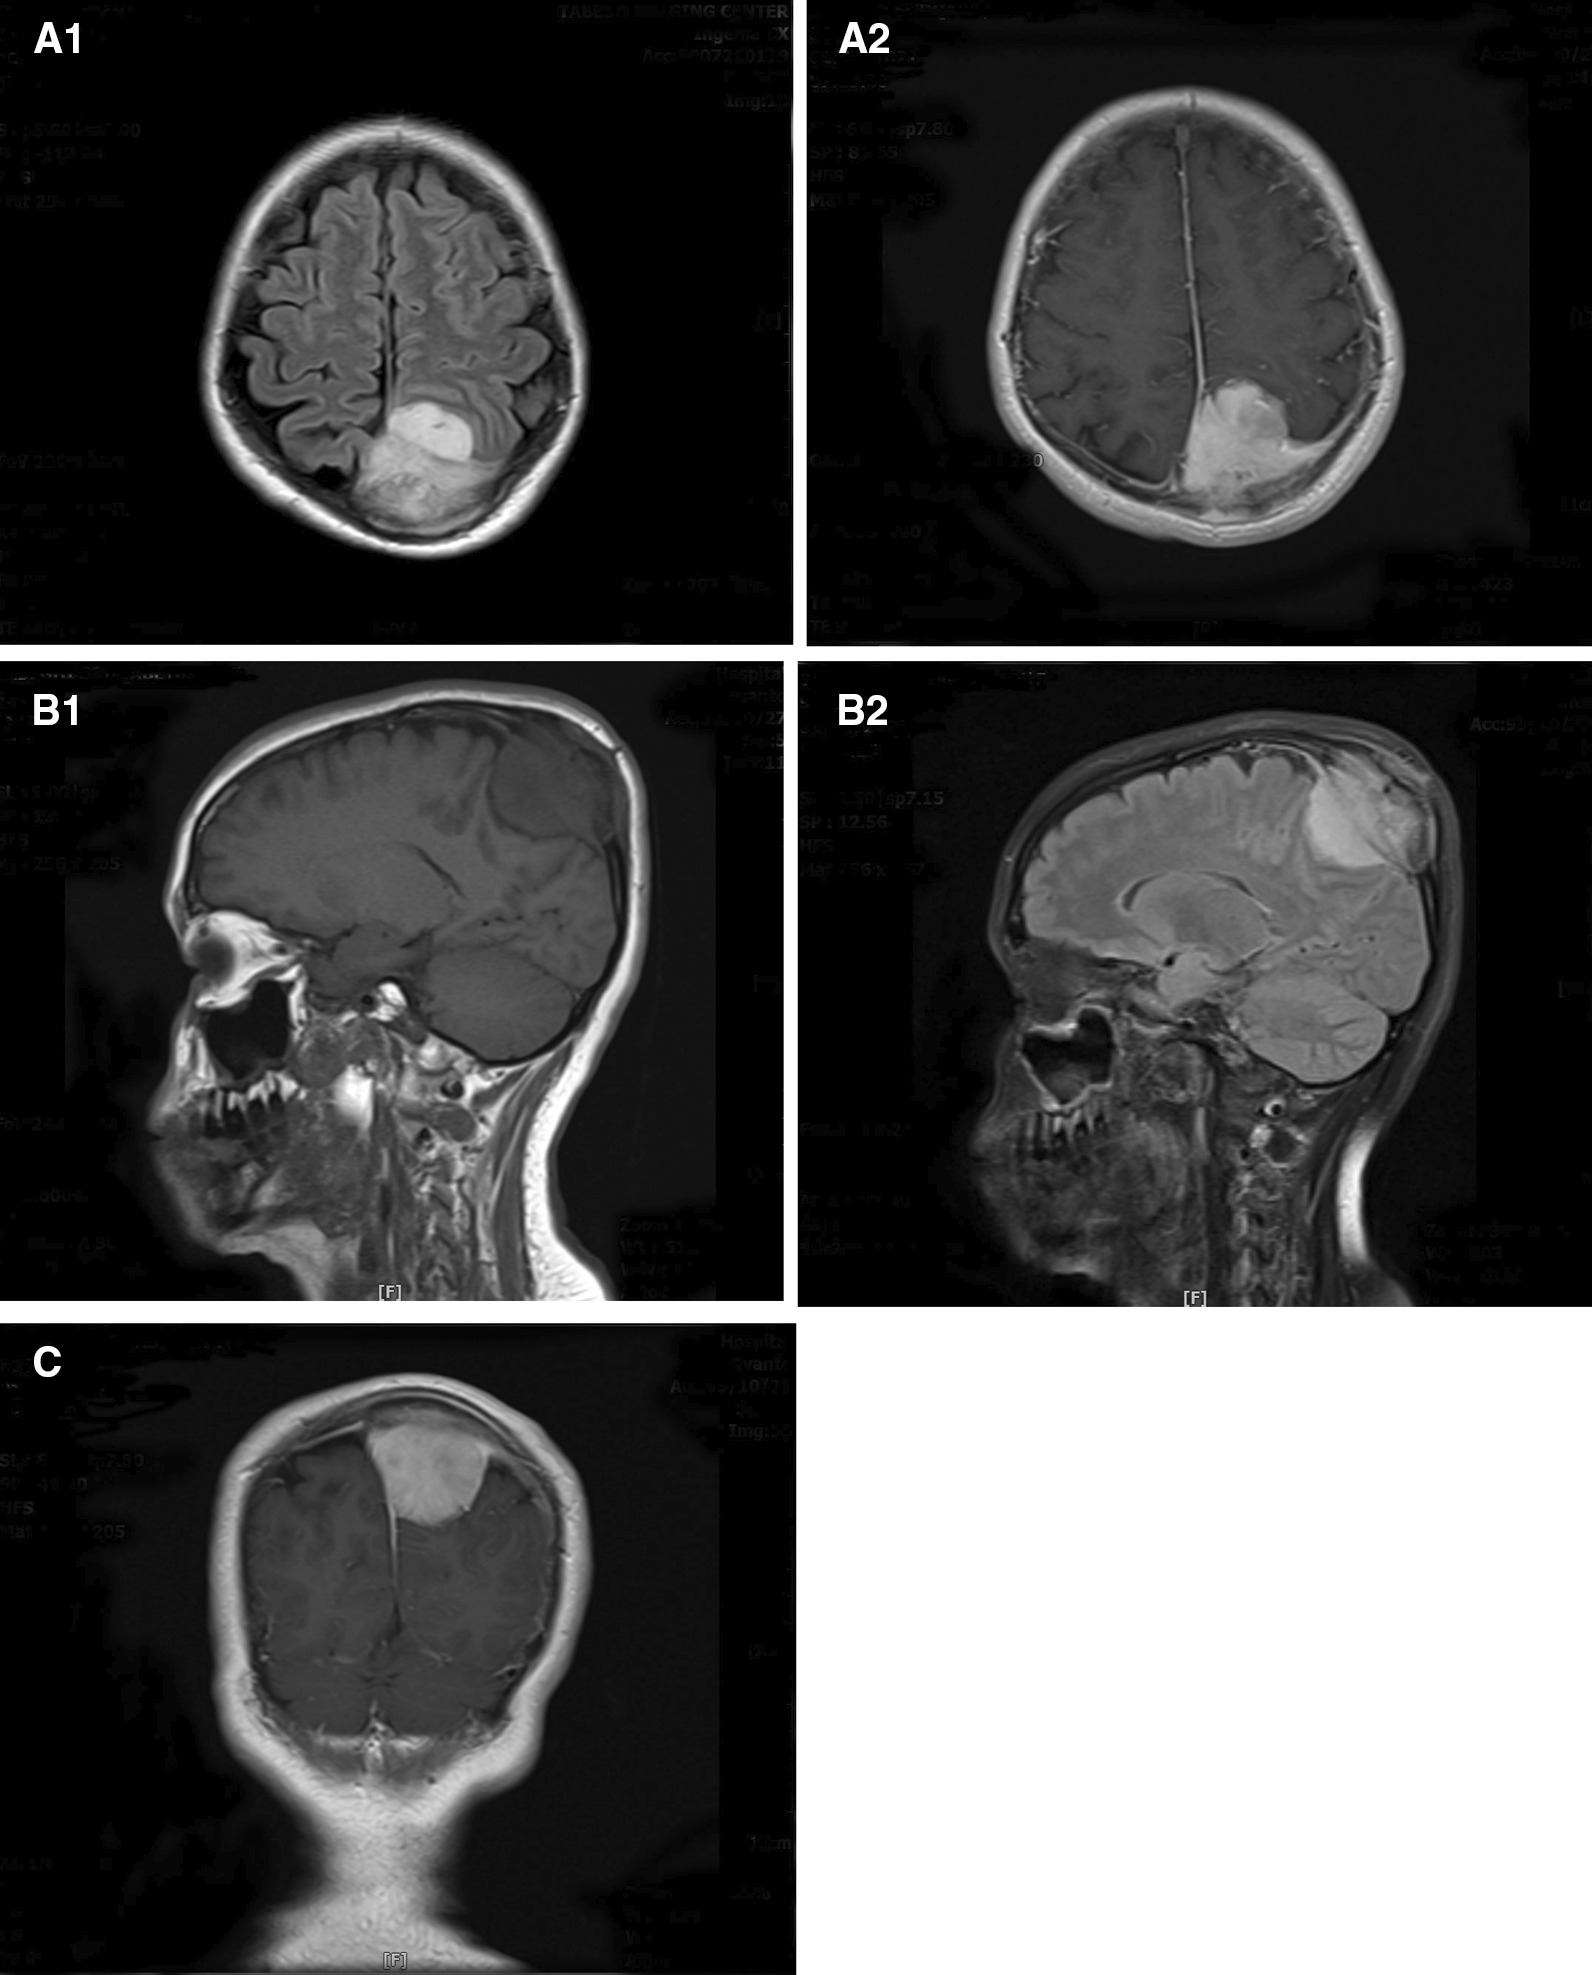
Preoperative magnetic resonance imaging of the patient with intracranial meningioma, showing a homogeneously enhancing tumor in the parasagittal region: (A1, A2) axial, (B1, B2) sagittal, and (C) coronal views
